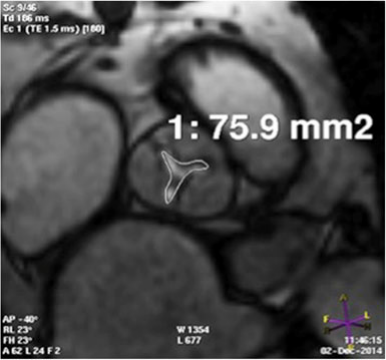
Assessment of aortic stenosis by planimetry. 2-D cine SSFP acquisition parallel to the valve plane demonstrates the narrowest opening of the aortic orifice. CT of the same patient also demonstrated similar orifice area
